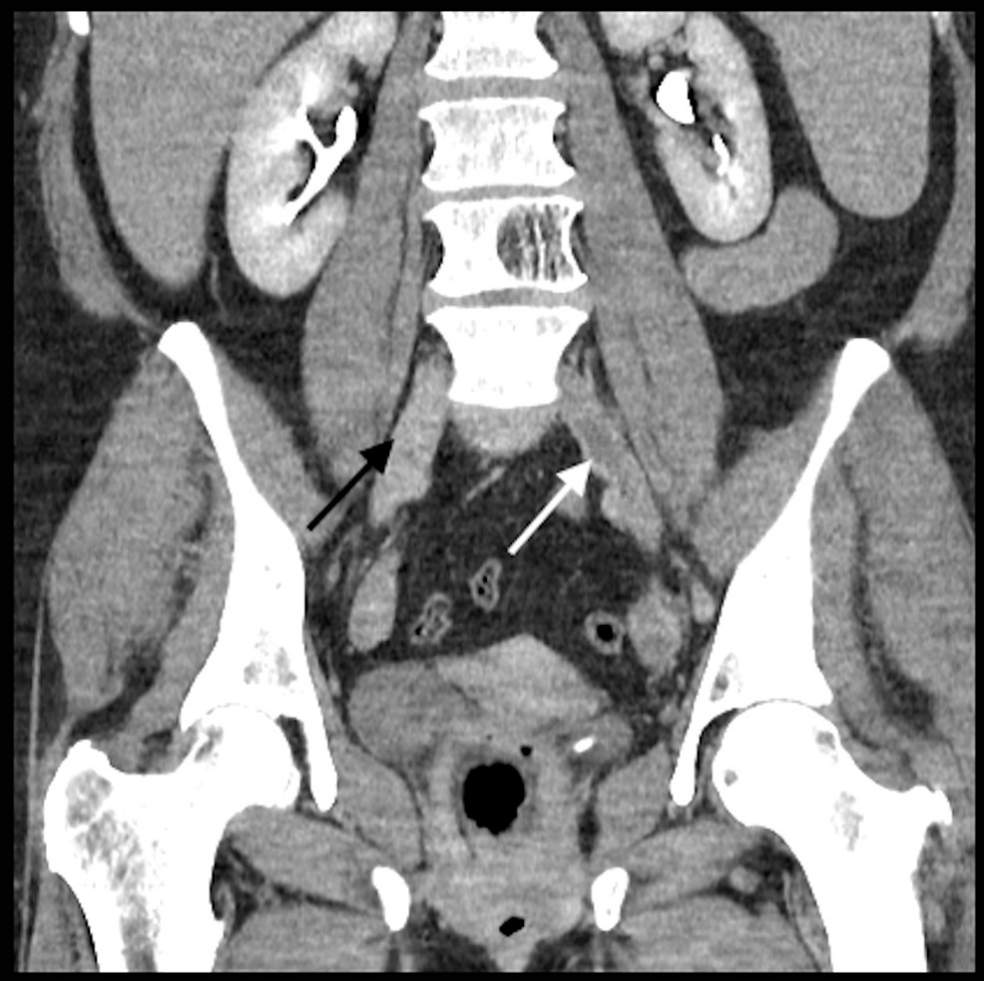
Scan on admission – CT venogram (coronal view) White arrow: thrombus in the left common iliac vein; black arrow: patent right common iliac vein
